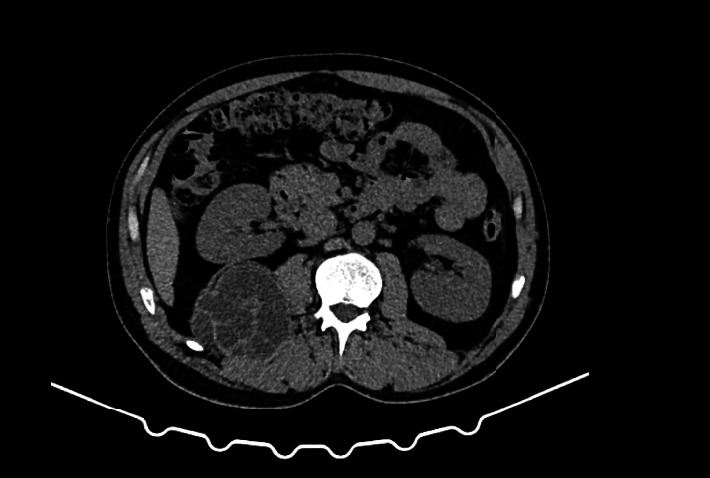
CT image of a retroperitoneal hydatid cyst on the right site.
